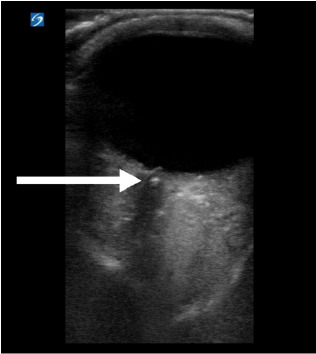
Point-of-care ultrasound demonstrating a central retinal artery occlusion. The arrow indicates the retrobulbar spot sign within the optic nerve sheath.
